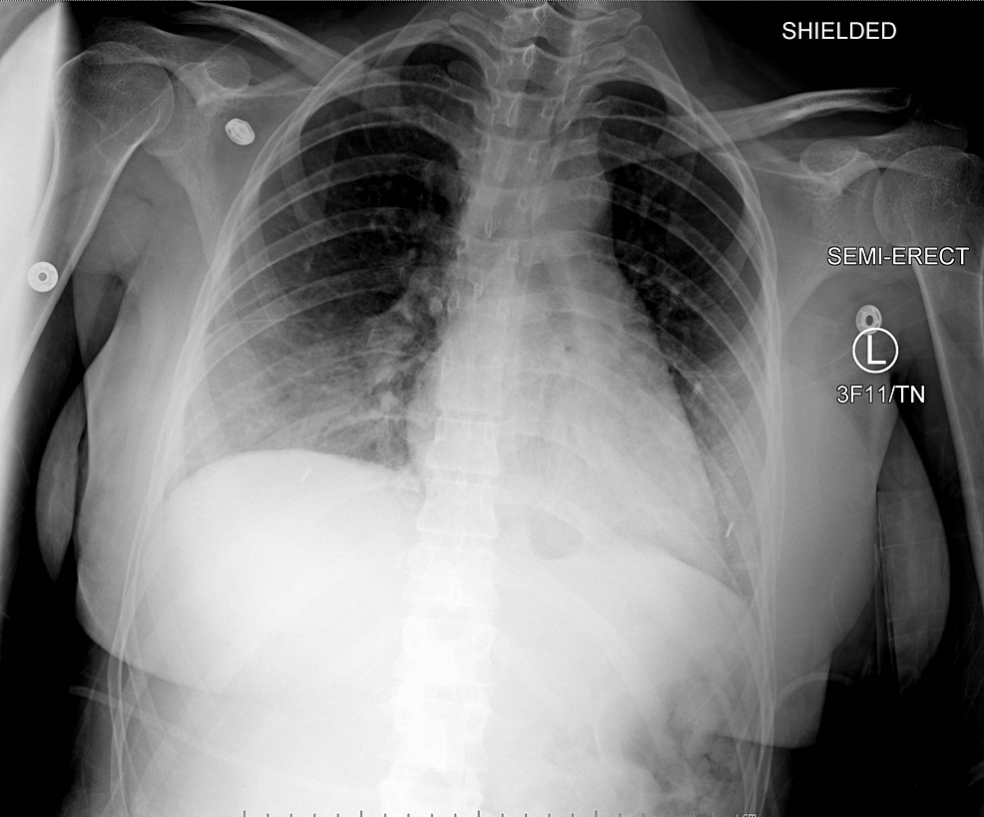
Chest radiograph on day of admission L: left
